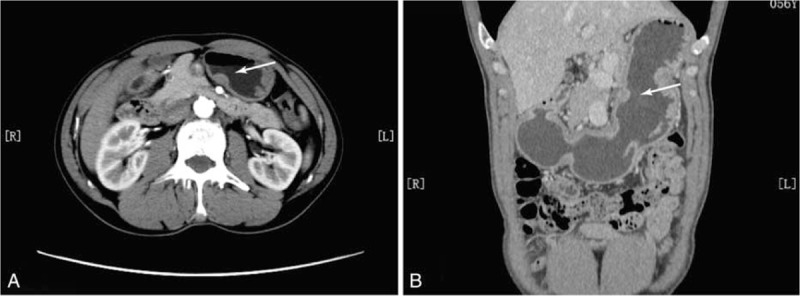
The abdominal enhanced CT scan indicated a nodule with similar density as soft tissue on the lesser curvature of the stomach (A). The CT coronal reconstruction displayed a submucosal tumor on the small curved side of the middle stomach region (B).
